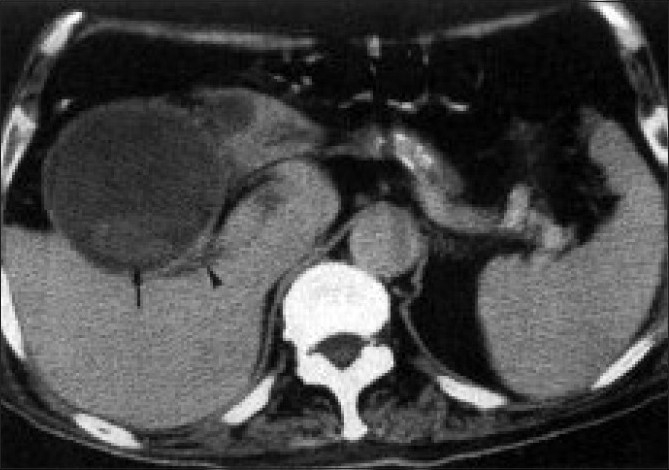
Contrast CT scan showing gallstones (arrow) with gallbladder rupture and fluid localized at the gallbladder fossa (arrowhead), penetrating into the left subhepatic area.
